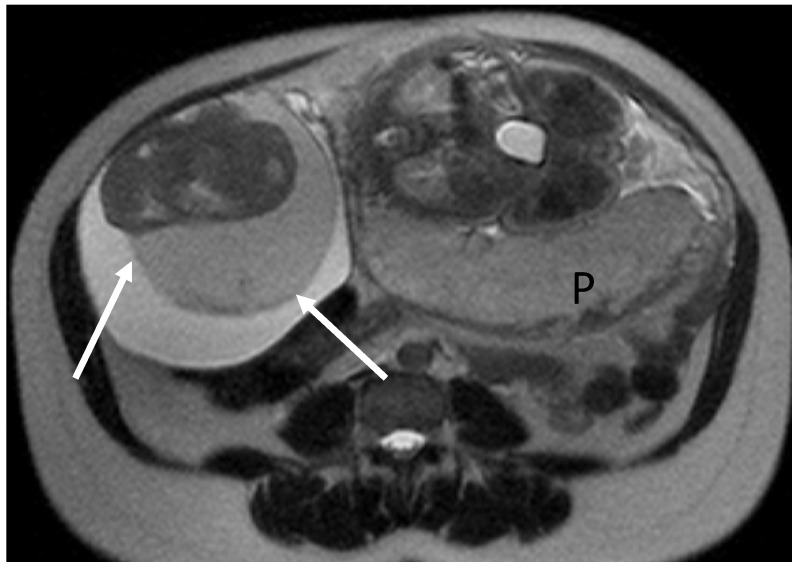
A 39-year-old pregnant woman at gestational week 30 presented with acute abdominal pain. Axial T2-weighted image demonstrates a large complex mass attached to the right uterine side (arrows). A mucinous cystadenocarcinoma of the right ovary was found during surgery. P: Placenta.
